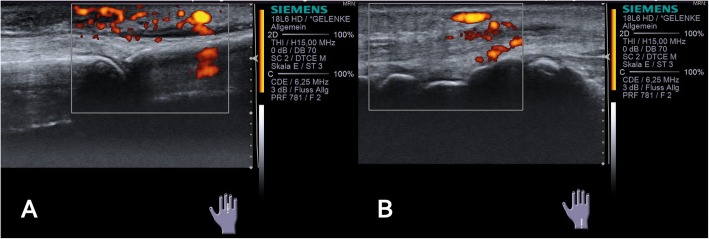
Ultrasound images of the third metacarpophalangeal joint. a Longitudinal view of the third metacarpophalangeal joint showing intense power doppler signaling III°. b Longitudinal view of the carpal bones demonstrating tenosynovitis of the fourth extensor tendon sheath with power doppler signaling III°
